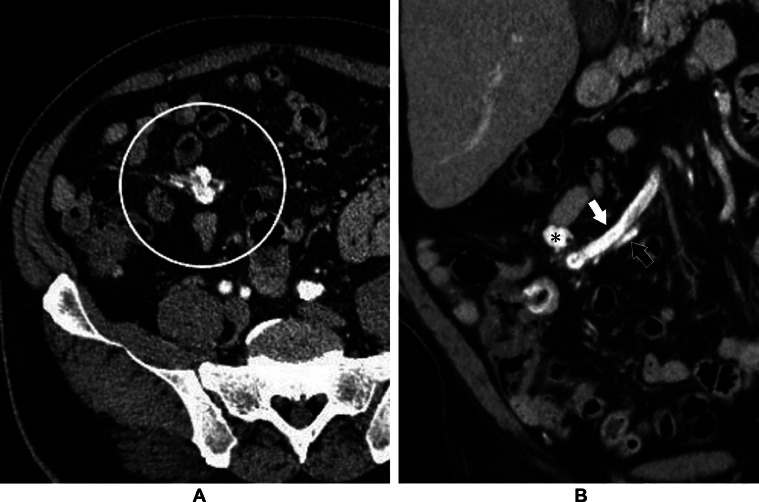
Abdominal contrast-enhanced computed tomography, early phase. (A) Axial image. An irregular, dilated vascular structure is visible in the mesentery (white circle). (B) Coronal image. The ileal artery (black arrow) connects with the dilated ileal vein (white arrow) via the dominant outflow vein (asterisk). Contrast enhancement is visible in the ileal vein at about the same level as in the ileal artery, leading to the diagnosis of an arteriovenous malformation.
